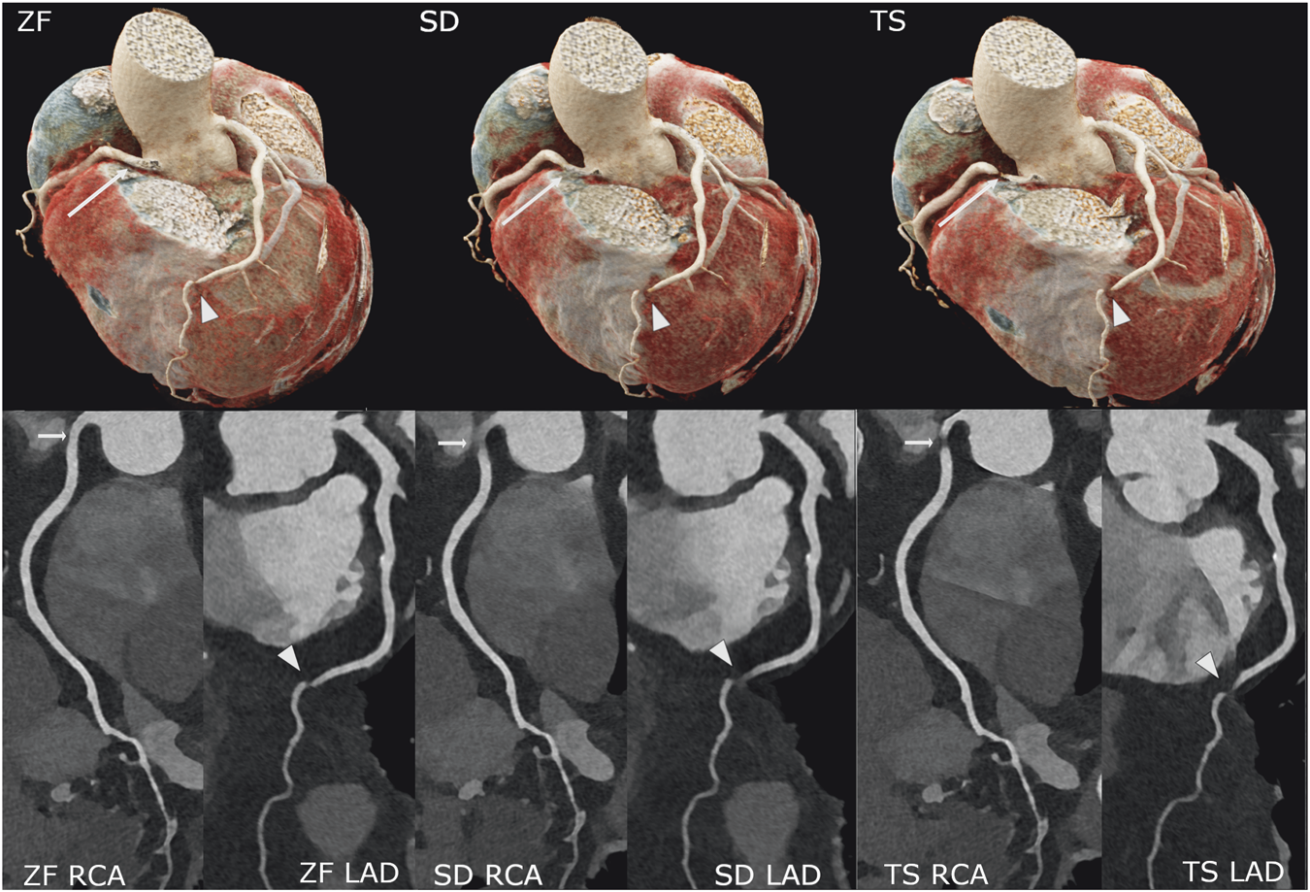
Coronary CT angiography (cCTA) of a 65-year-old female patient was performed for the evaluation of coronary artery disease. The scan was conducted in sequential UHR mode at an average heart rate of 71 bpm, with the administration of 15 mg of beta-blocker and 0.8 mg of nitroglycerin. The upper row displays the 3D heart reconstruction images based on the different reconstruction algorithms: ZeeFree (ZF), Standard (SD), and TrueStack (TS). The bottom row shows curved planar reformations of the right coronary artery (RCA) and the left anterior descending artery (LAD), including the left main coronary artery, for the different reconstruction algorithms. The artifact in the distal LAD (arrowhead) is prominent in TS and SD images but diminished in the ZF image. The arrow indicates a severe artifact in the proximal RCA on TS images, which is reduced in SD images, although the vessel cannot be confidently assessed. In the ZF image, the RCA is displayed in excellent quality without any image artifacts
